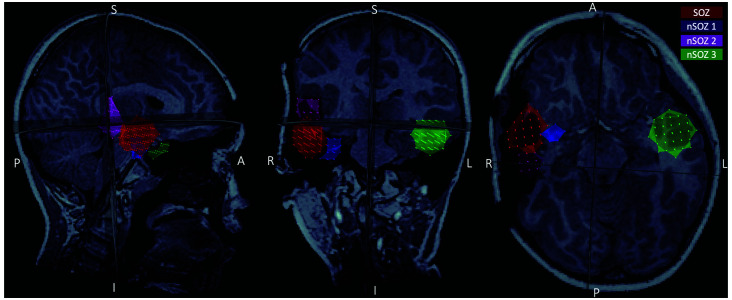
Postoperative MRI showing the four regions of interest (ROIs) used in the present study: the seizure onset zone (red), two ROIs in the resected region but outside the seizure onset zone (SOZ; pink and blue), and a homologous ROI contralateral to the SOZ (green).
